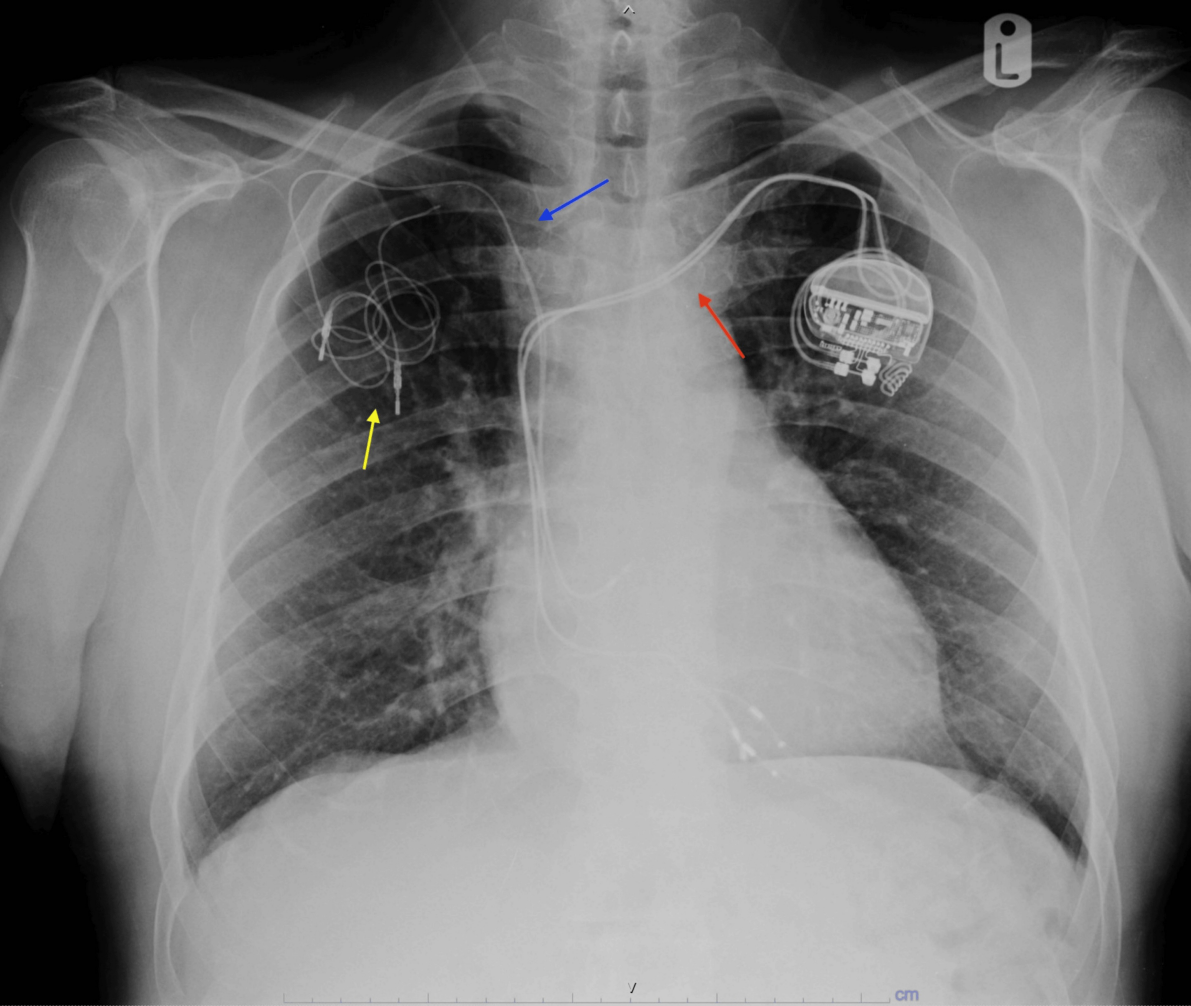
Chest X-ray showing abandoned right-sided leads and newly implanted left-sided pacing leads. The right ventricle failed lead extraction is indicated by the coiled lead (yellow arrow). Abandoned right ventricular leads are shown (blue arrows). The new dual-chamber device features right atrial and right ventricular leads passing through the left brachiocephalic vein (red arrows).
